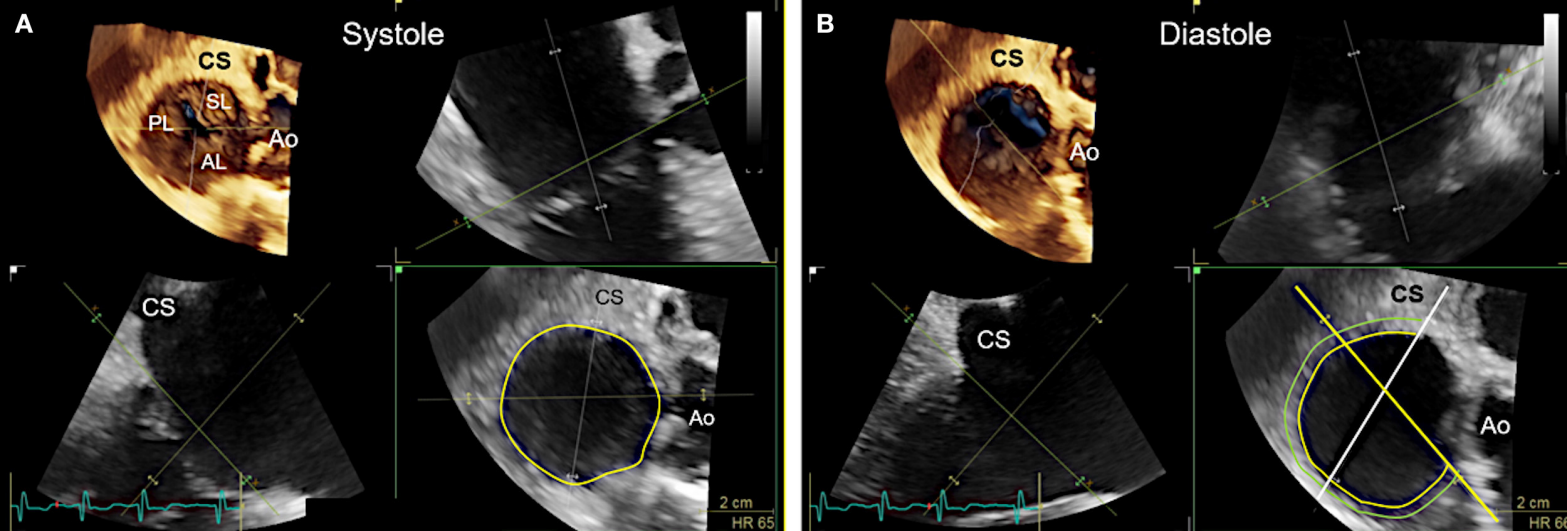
Measurement of tricuspid annular dimensions in 3D transesophageal echocardiography (TEE) by using real-time multi-planar reconstruction (RT-MPR). (A) The 3D data set is acquired from a mid-esophageal 60? RV inflow-outflow tract view. RT-MPR is used to measure the annular circumference (yellow circle; bottom right) after the green cropping line is adapted to the annular plane in the orthogonal views (top right and bottom left). (B) Measurements for band sizing should be made in diastole, when annular dimensions are largest (51). The green cropping lines are adapted to the annular plane in the same way as shown in (A) (top right and bottom left). In the z-plane (bottom right), the white line is then positioned at the end of the coronary sinus (CS) whereas the yellow line is positioned at the antero-septal annulus by sparing out the aorta (Ao). The hinge line (curved yellow line) is then traced in between the white and yellow line. A second line (light green) is drawn at about 4 mm away, where anchors can realistically be placed in tissue. This measurement is used to confirm the pre-selected band size which was obtained from multi-detector computed tomography imaging measurements.
